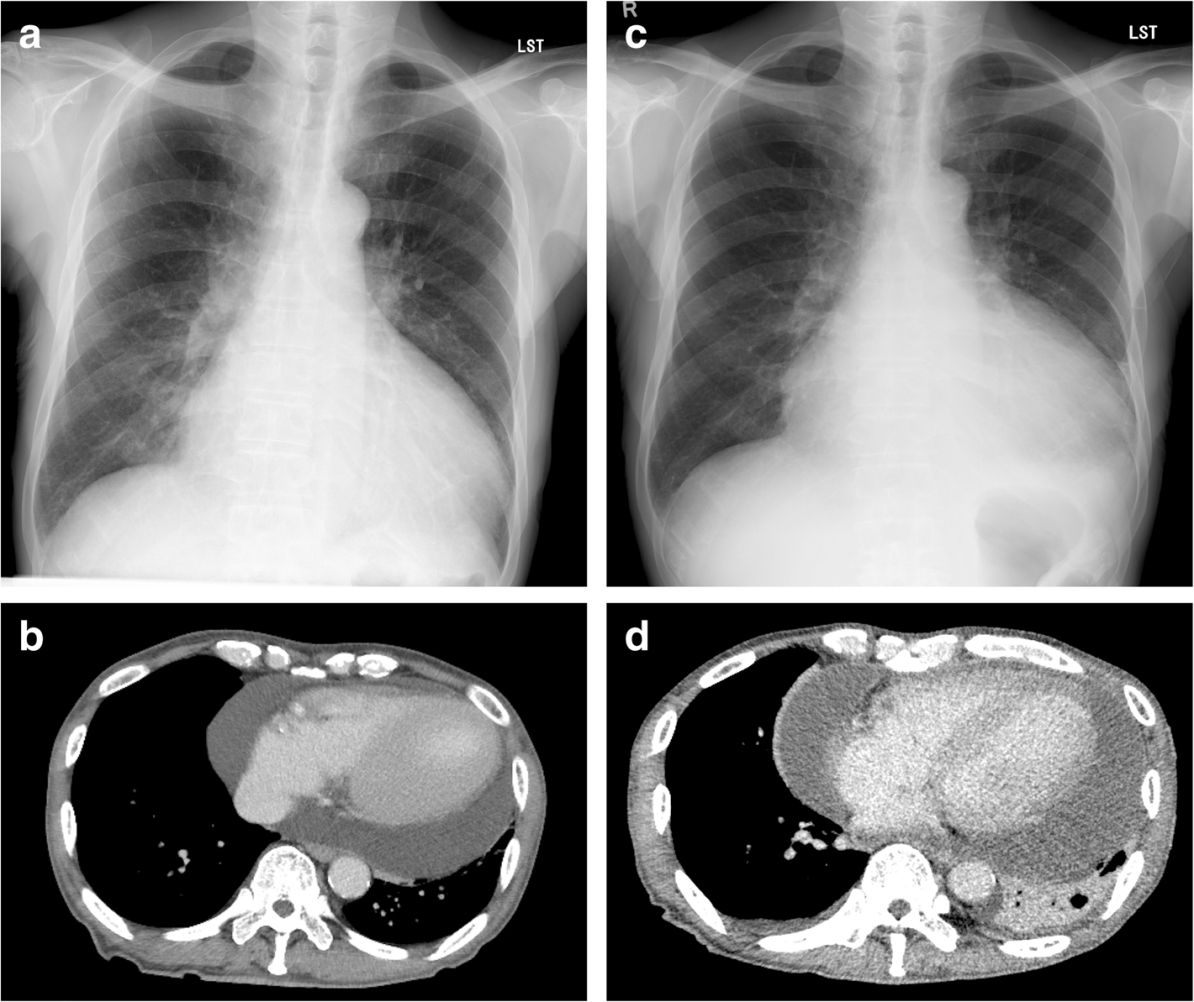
Severe cardiomegaly revealed on chest radiography at the initial outpatient appointment (a). Chest computed tomography showed a large pericardial effusion with an estimated volume of 700 mL (b). Cardiomegaly worsened 4 weeks after the initial appointment (c). Chest computed tomography showed a huge pericardial effusion with an estimated volume of 1300 mL (d)
